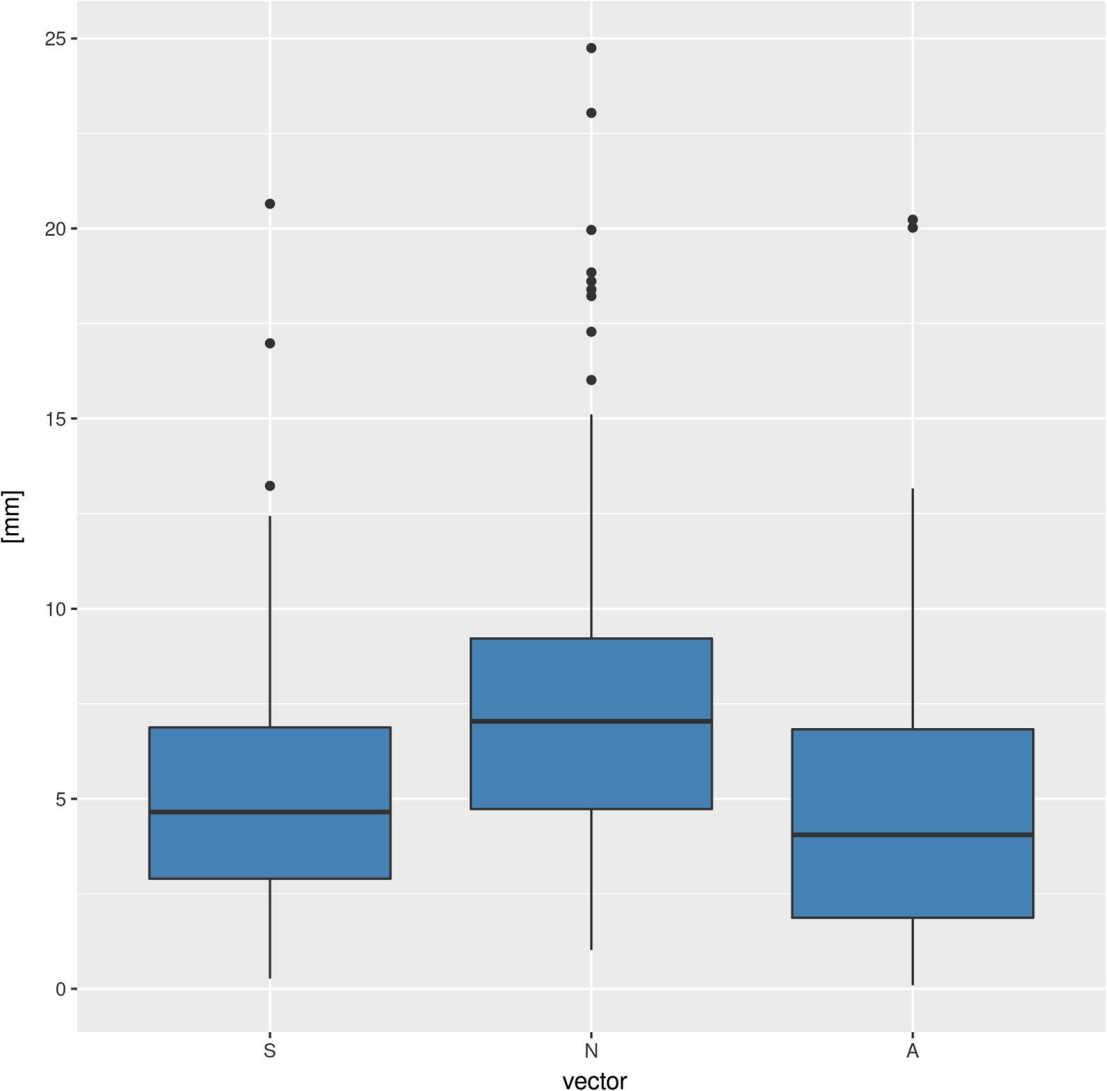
Length of displacement vectors A, N and S describing differences in location between the reference points between the two radiographs CEPH_I_ and CEPCH_II_
